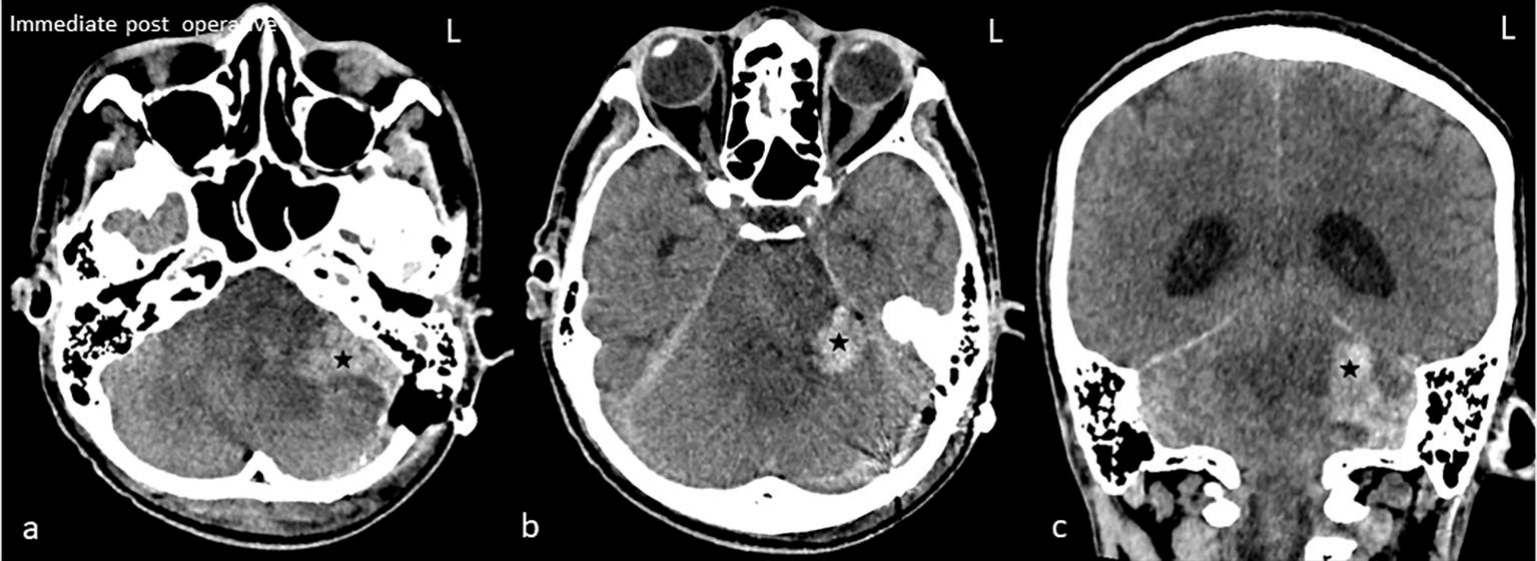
Immediate post-op plain computed tomography of the brain in axial (A, B) and coronal (C). Hyperdense hematoma (star) at the left cerebellopontine angle is seen. The fourth ventricle is obliterated with resultant hydrocephalus.
